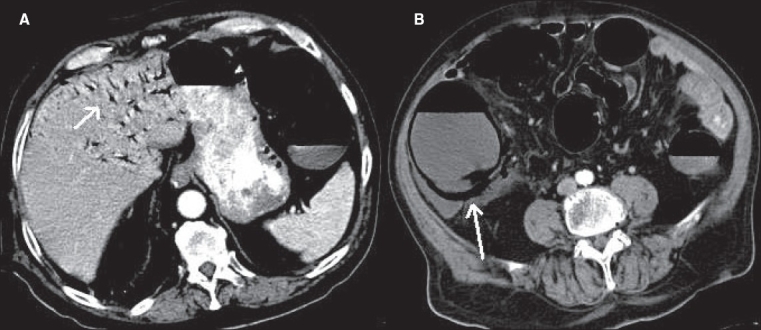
Bowel infarction with perforation. Contrast-enhanced CT shows portal venous air (short arrow in A) and bowel pneumatosis (long arrow in B) with dilated bowel loops
